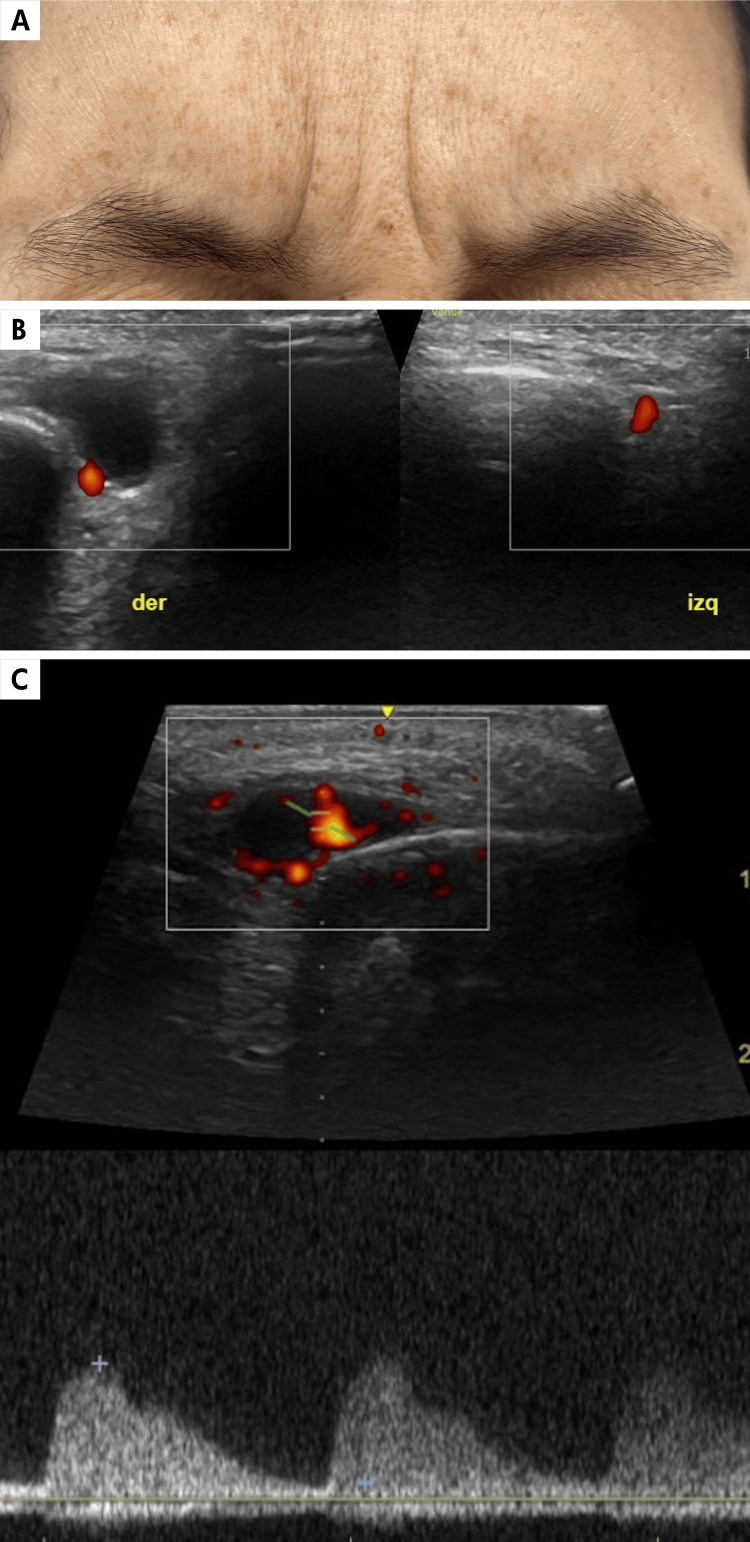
Pseudoaneurysm seen with high-resolution gray-scale and Doppler color duplex ultrasound. A. Clinical image of a patient with a sensation of right supraorbital mass becoming more evident with facial contraction. B. Comparative axial gray-scale and Doppler color duplex ultrasound image of the supraorbital region. The right and left supraorbital arteries are normal. On the right side, above the artery, there is an anechoic, oval-shaped, pseudo-cystic image (delimited by calipers +). C. Spectral waveform confirming arterial flow within the lesion. Corresponds to a pseudoaneurysm.
